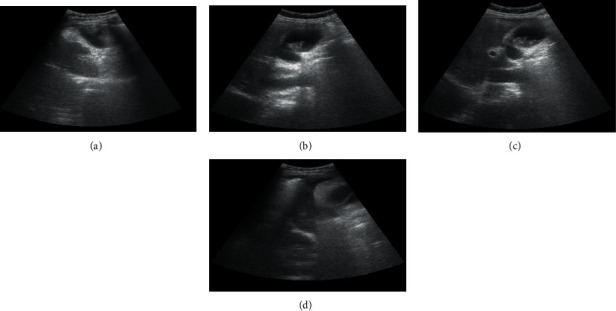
Conventional ultrasound examination results. (a) Nodular type, with moderate and weak echoes. (b) Thick-walled type, with diffuse and uneven thickening of the gallbladder wall. (c) Solid type, with an enlarged gallbladder, strong echo group with sound shadow. (d) Mixed type, the gallbladder wall was thickened regularly with papillary protrusions.
